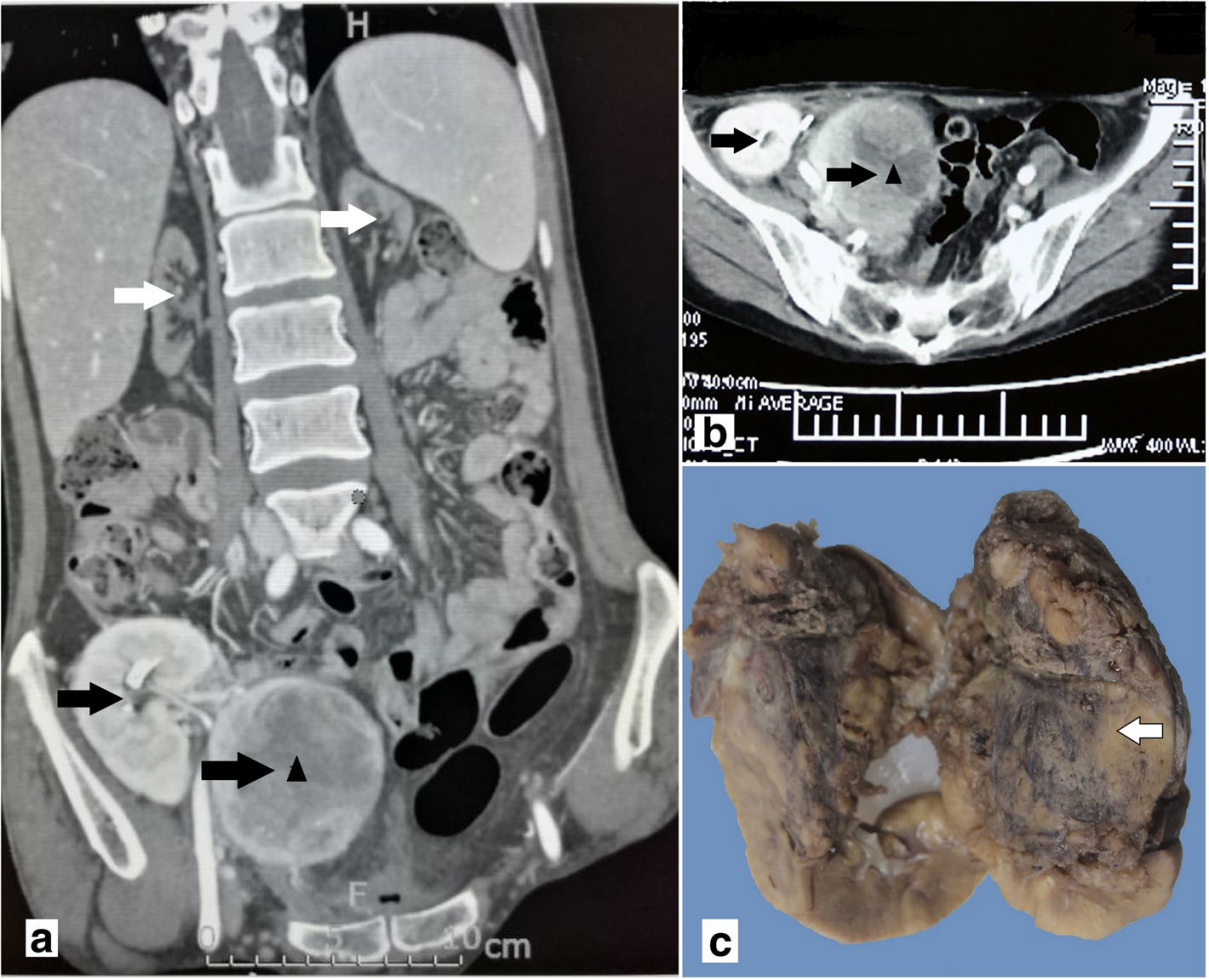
Computed tomography (CT) scan revealed that both original kidneys exhibited atrophy (a, white arrows) and a large mass (a and b, triangle) measuring 7.4 × 6.3 × 6.0 cm with a mixed density in the upper pole of the inner transplanted kidney (a and b, black arrows). c. Macroscopic features of MRT of the transplanted kidney. The cut surface of the mass was white to grayish (arrow)
